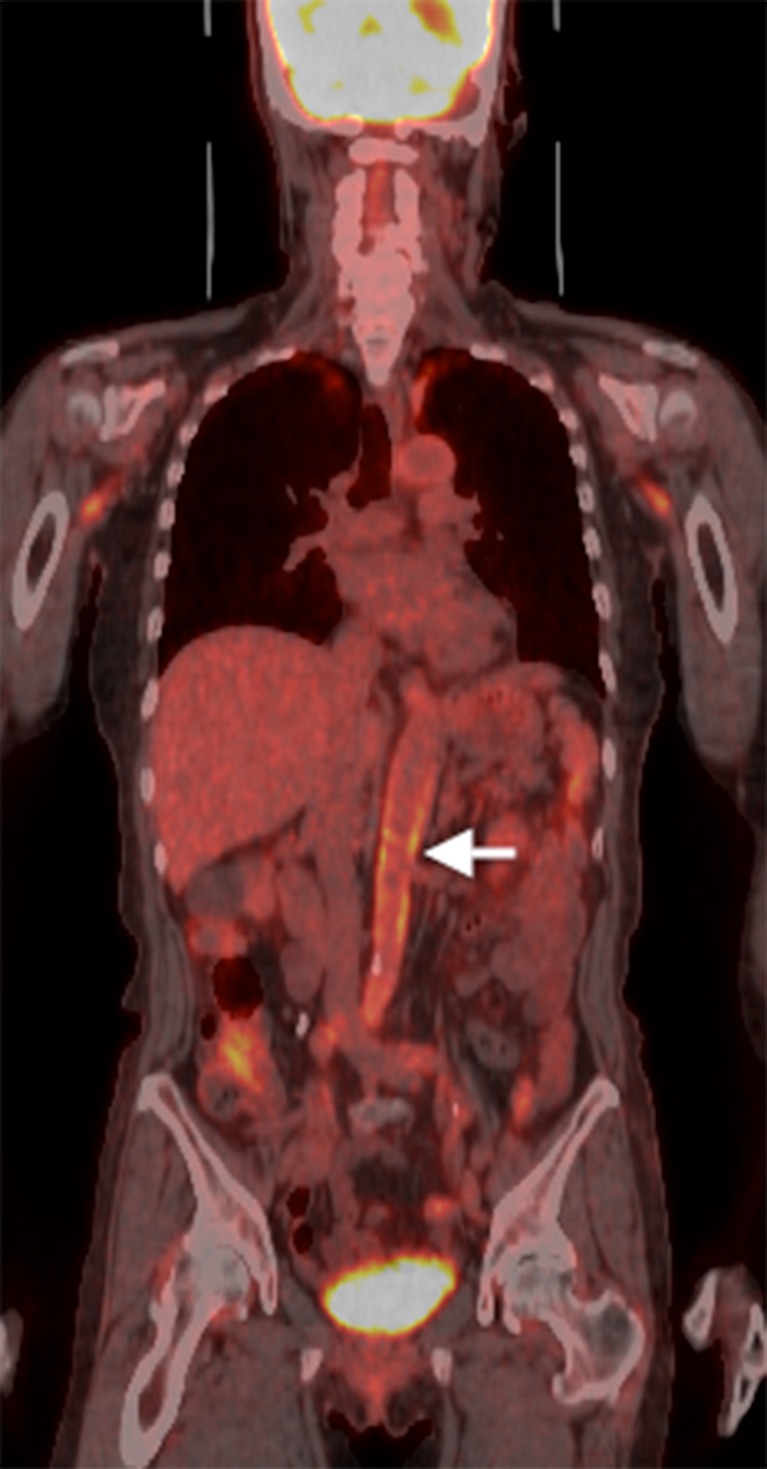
FDG-PET image with an arrow pointing to abnormal uptake in the abdominal aorta.
